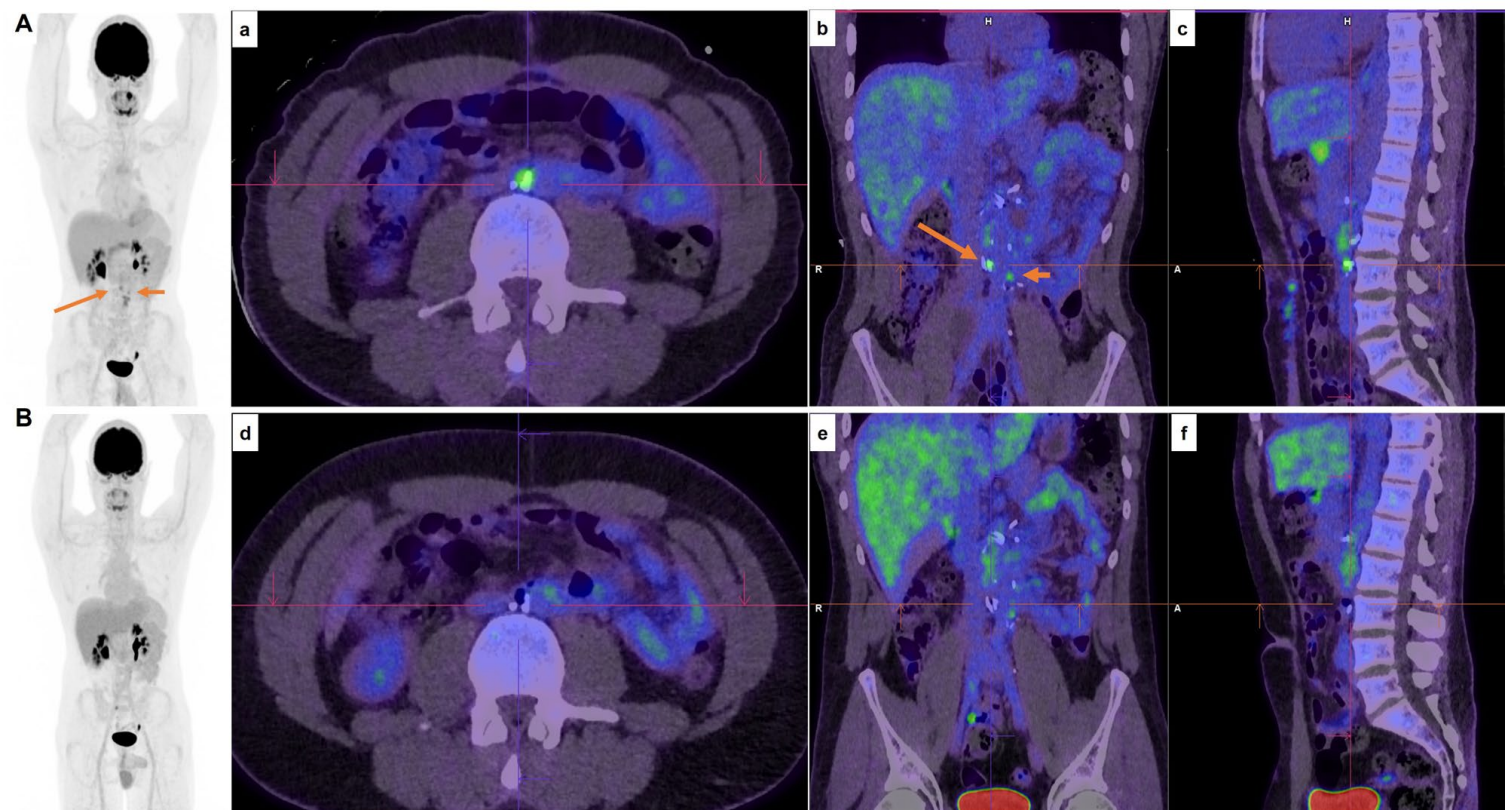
A 33-year-old male with a history of non-seminoma germ cell tumor, previously treated with left orchidectomy, chemotherapy, and retroperitoneal lymph node dissection for bulky para-aortic lymph node metastases, presents for a progress assessment. The PET scan (Image A) demonstrates mild foci of uptake around the aortocaval (SUV max 3.9) and left para-aortic (SUV max 3.3) regions at the level of L3, adjacent to surgical clips (red arrows, a-c), which were reported as indeterminate (either post-surgical inflammatory changes or residual disease). Twelve months later, a follow-up PET scan (Image B) showed an interval reduction in the intensity of retroperitoneal foci of uptake (d-f), consistent with resolving post-operative inflammatory changes
